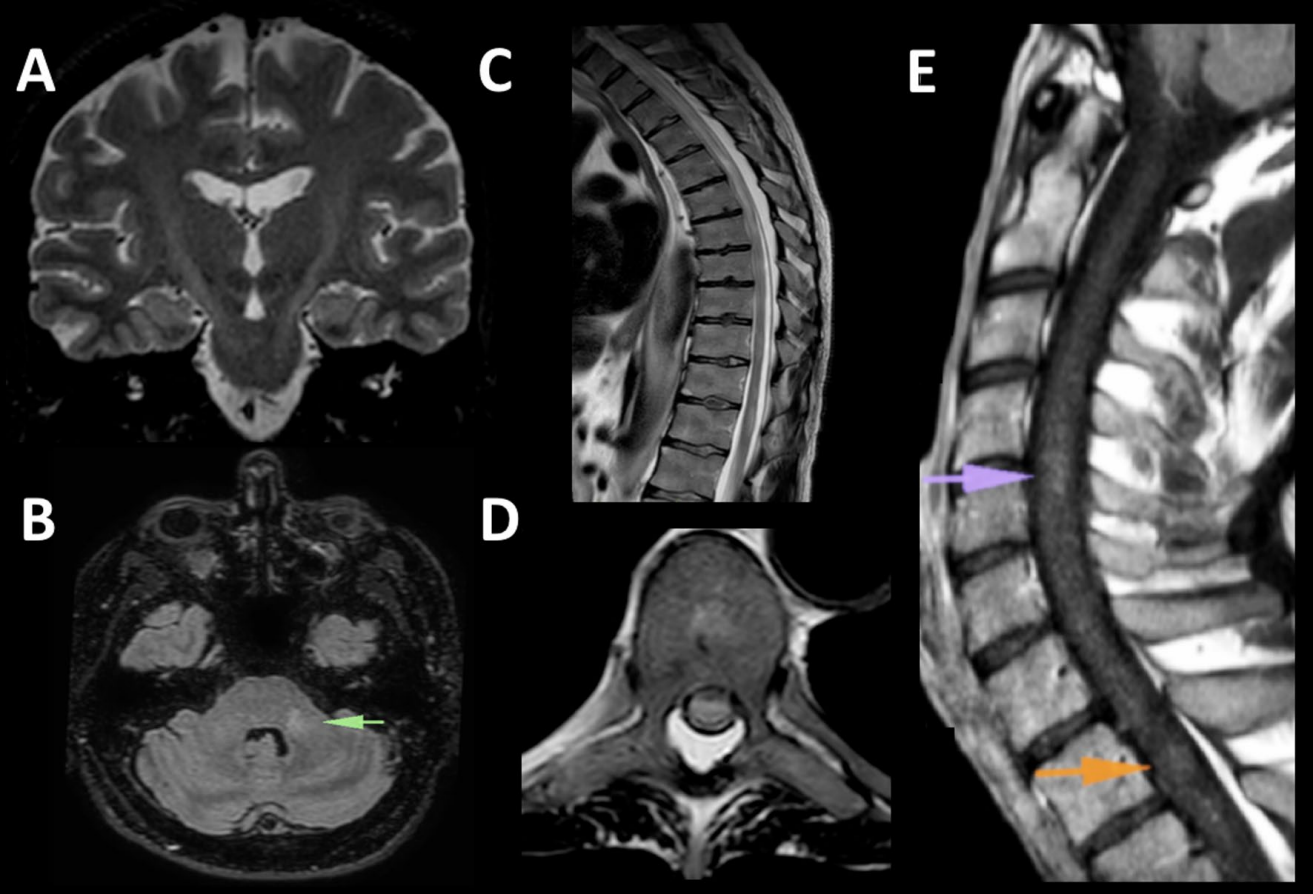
A Coronal T2-weighted MRI displays hyperintense signal along the corticospinal tracts bilaterally (“wine glass” sign). B On axial fluid attenuated inversion recovery (FLAIR), a hyperintense lesion is shown in the left middle cerebellar peduncle (green arrow). On brain MRI, no contrast enhancing lesions or lesions with diffusion restriction were depicted, while the optic nerves had normal appearance (images not shown). C Sagittal T2-weighted spine MRI shows longitudinally extensive thoracolumbar spinal cord lesions, with combined gray/white-matter involvement, affecting more than two-thirds of the thoracic spinal cord’s cross-sectional area on axial T2-weighted MRI sequence (D), findings compatible with transverse myelitis. E Sagittal T1-weighted gadolinium-enhanced MRI reveals contrast-enhancing lesions in the cervical and thoracic spinal cord (purple and orange arrows, respectively)
